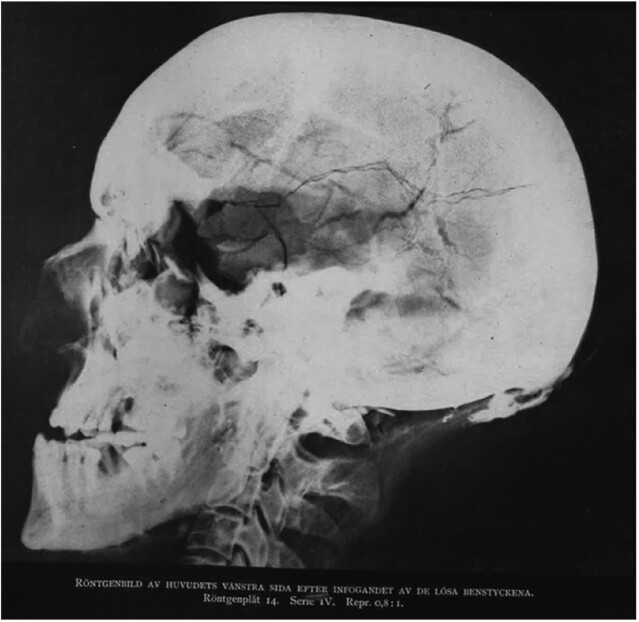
Radiographs from the latest autopsy performed in 1917 (1) show no traces of lead in the wound channel or elsewhere in the cranium.
